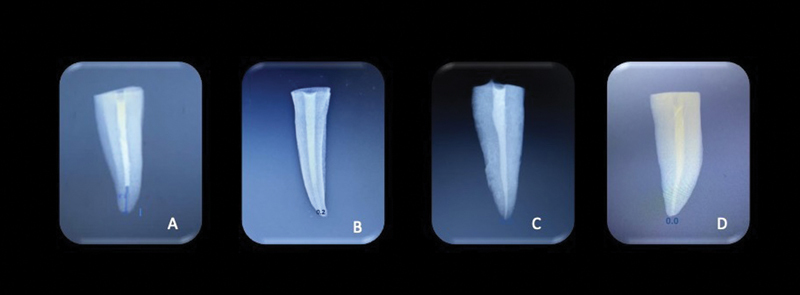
Digital radiographs of the teeth with dye penetration. (
